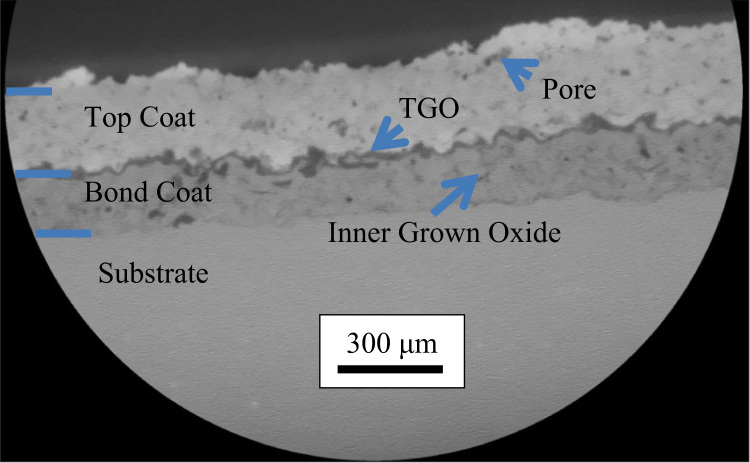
One slice from the 3D microstructure observed by CT, top coat, bond coat, TGO and substrate can be distinguished; some large pores and inner grown oxide can also be observed.
